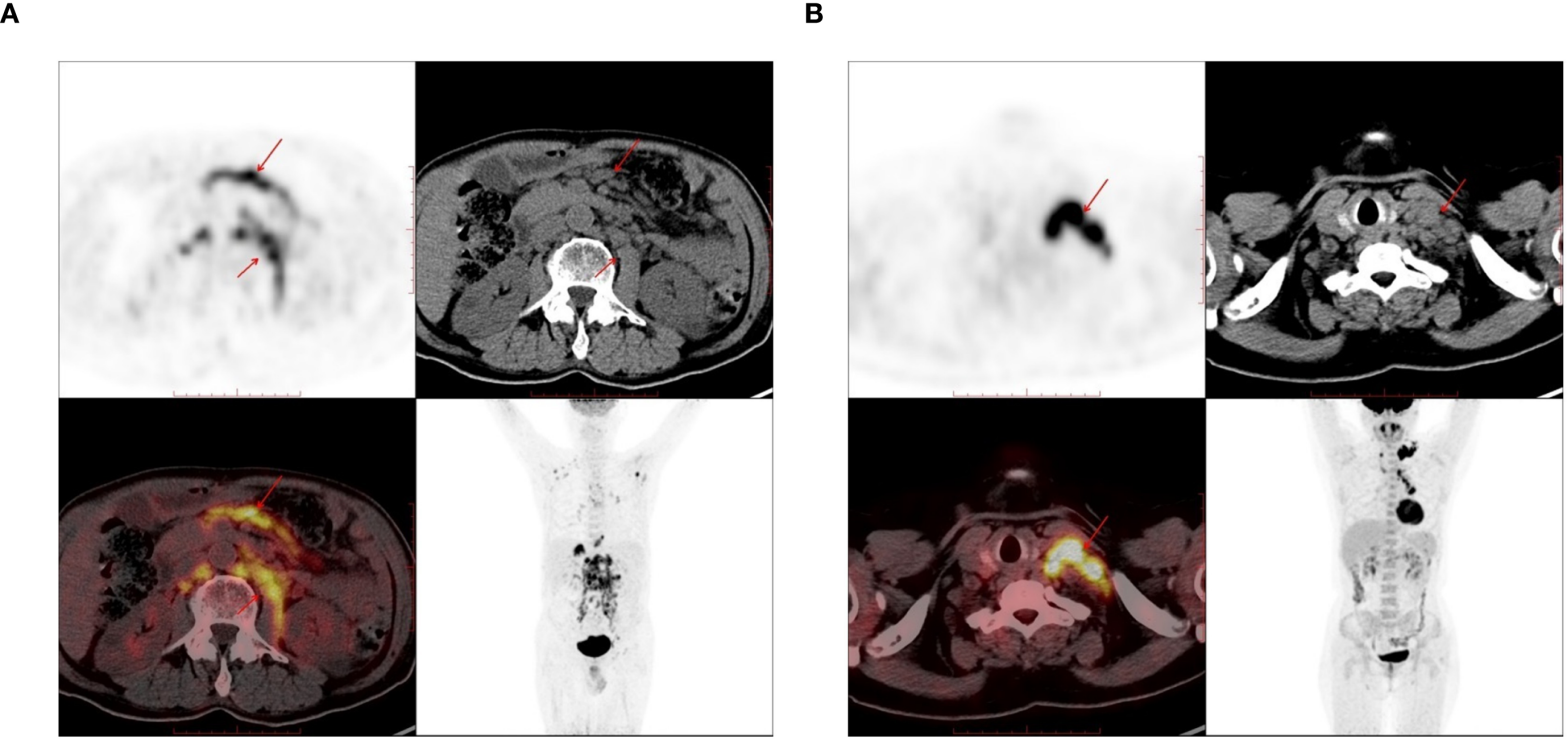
The PET-CT images used in the study. (A) 72-year-old male, there are enlarged lymph nodes in the abdominal cavity and retroperitoneum, which were pathologically diagnosed as diffuse large B-cell lymphoma (DLBCL). (B) A 61-year-old female, there are enlarged lymph nodes in the left supraclavicular region, which was pathologically diagnosed as lung adenocarcinoma.
